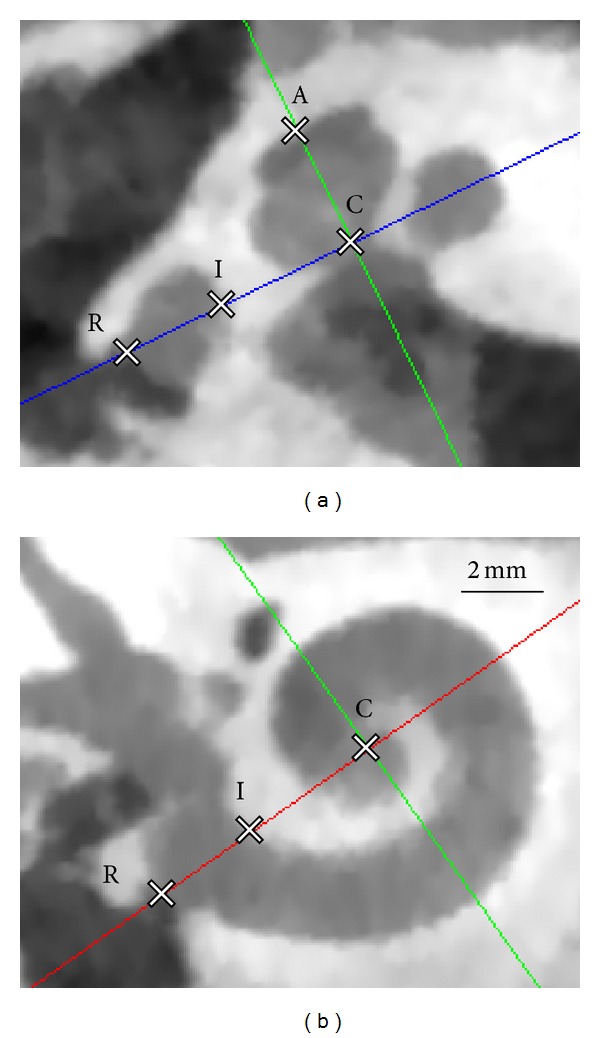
Landmark identification of a right human cochlea using cone beam CT data. (a) Oblique axial slice corresponding to the 0° reference plane (red line in (b), as defined in [18]). The RW center adjacent to the bony overhang (R), the inner wall border at the RW (I), the center of the modiolus in the basal turn (C), and the apical center of the modiolus (A) are used to define a local cochlear coordinate system for further computations. (b) Oblique coronal slice of the basal turn (blue line in a) and corresponding in-plane landmark positions.
